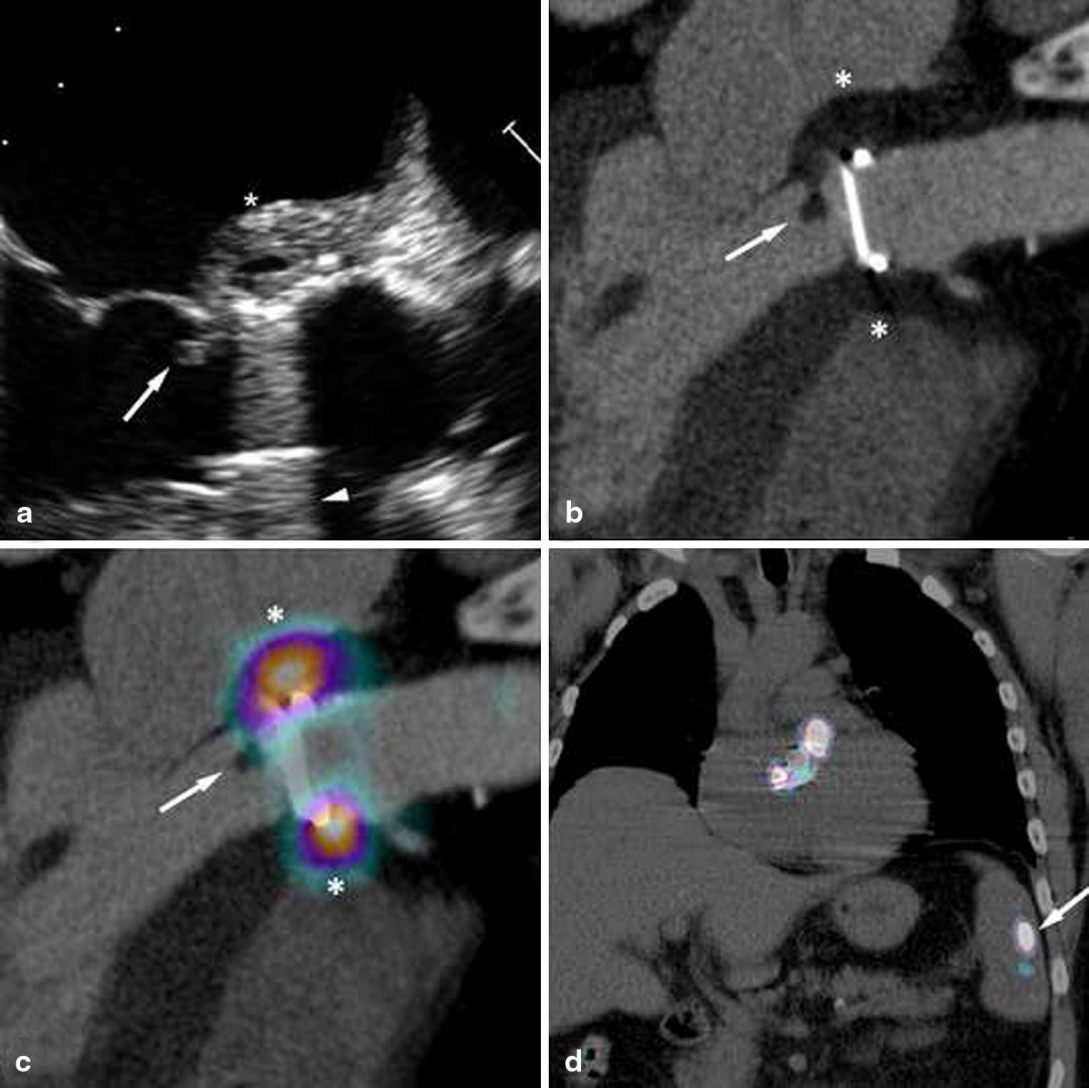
Metastatic infection in PHV endocarditis detected by whole body FDG-PET/low-dose CT. Bileaflet mechanical PHV in aortic position for 6 years, and seven consecutive blood cultures with Streptococcus pneumoniae. a TEE showed a vegetation (*arrow*) and thickened wall (*asterisk*) without colour Doppler flow in the former non-coronary cusp region, suggestive of an abscess. Former right coronary cusp (RCC) imaging is hampered by reverberations (arrowhead). b CTA confirmed TEE findings but also detected an abscess in the RCC region (*asterisks*). Panel c: CTA fused with FDG-PET confirmed abscesses (*asterisks*). The vegetation (*arrow*) did not show FDG uptake. Additionally, whole body FDG-PET/CT showed a metastatic infection in the spleen (Panel d, *arrow*), in this case an abscess requiring percutaneous drainage. Re-printed with permission [20]
